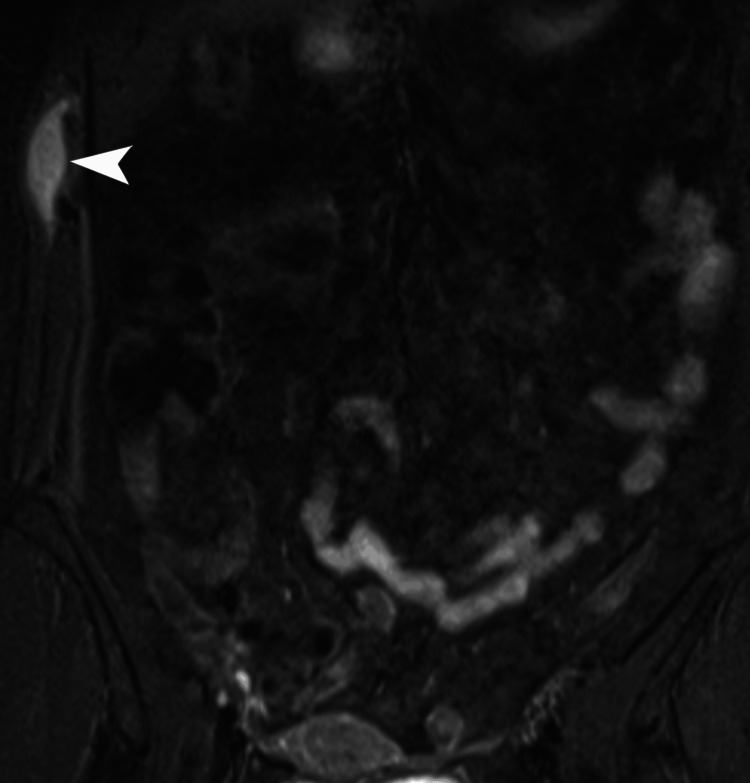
MRI of the right chest wall cystic lesion. Coronal MRI of the chest and abdomen demonstrates a T2-hyperintense lesion with fluid signal intensity along the right lateral abdominal wall (white arrowhead). MRI: magnetic resonance imaging
